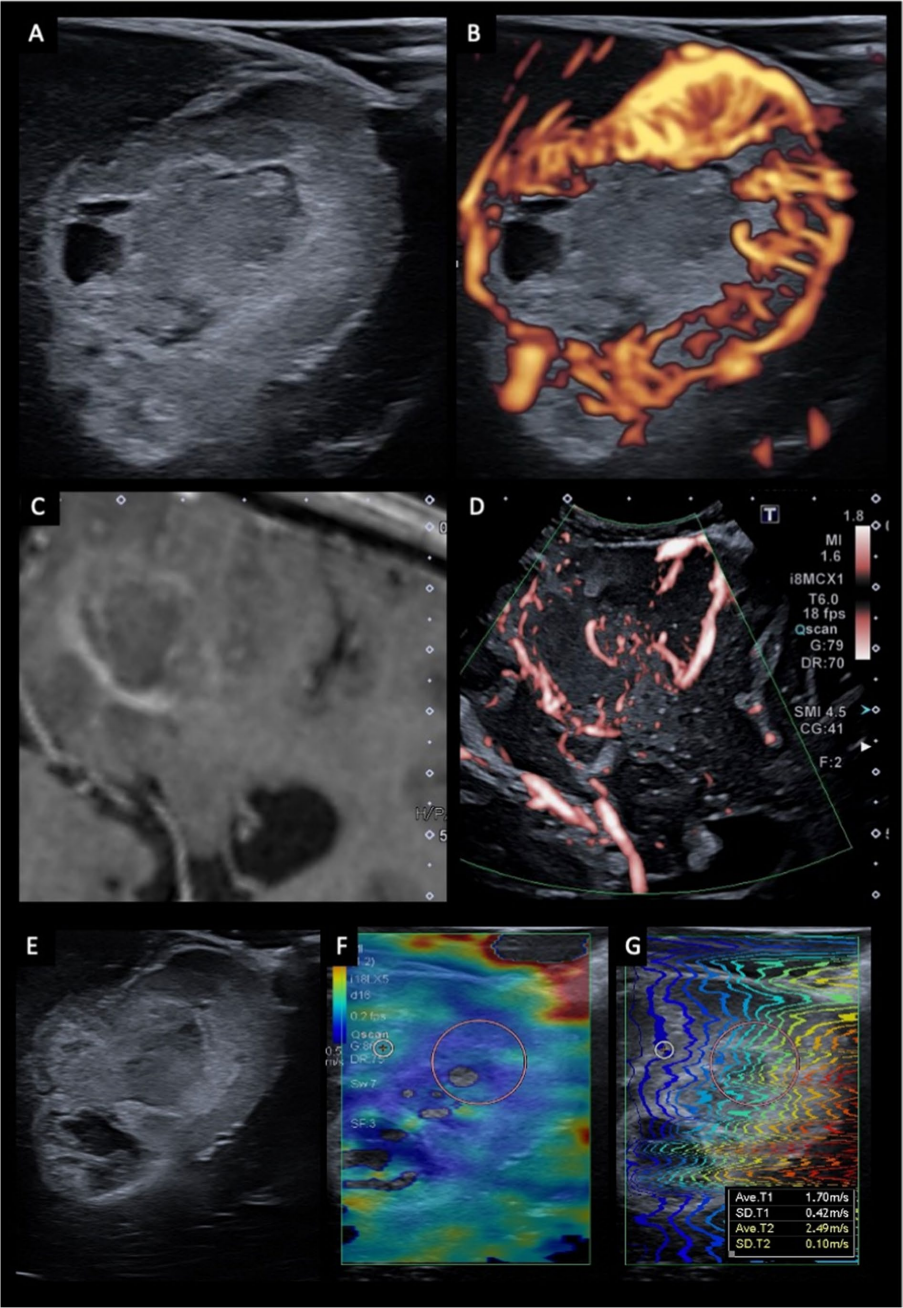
Advanced ultrasound imaging techniques. Examples of US doppler using superb microvascular imaging (SMI) (A-D) and elastography (E–G). B-mode (A) and doppler (B) imaging of a glioblastoma shows a relatively well-circumscribed, hyperechoic tumor with small hypoechoic areas in keeping with cystic necrosis (A). The tumor exhibits marked peripheral hypervascularity in keeping with neoangiogenesis (B). Post-contrast T1-weighted MRI (C) and doppler imaging (D) of a glioblastoma demonstrates the ability of US doppler imaging to resolve and identify small pial and parenchymal vessels (red) that are not appreciable on MRI. B-mode US imaging (E) and shear wave elastography (F, G) of a glioblastoma. The tumor demonstrates reduced stiffness compared to the surrounding brain, which is reflected by a heat map with blue reflecting low stiffness and red high stiffness
